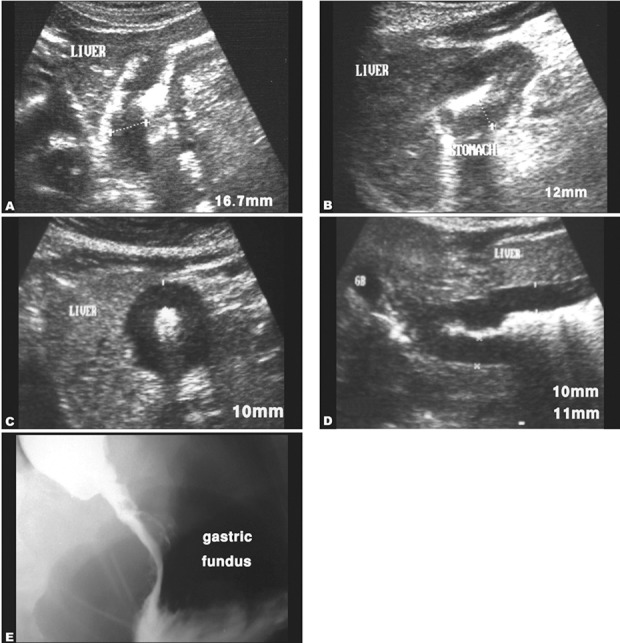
Abdominal ultrasound images showing gastric adenocarcinomas in fundus A, body B and antrum C,D of the stomach. E. The barium meal image of case A
